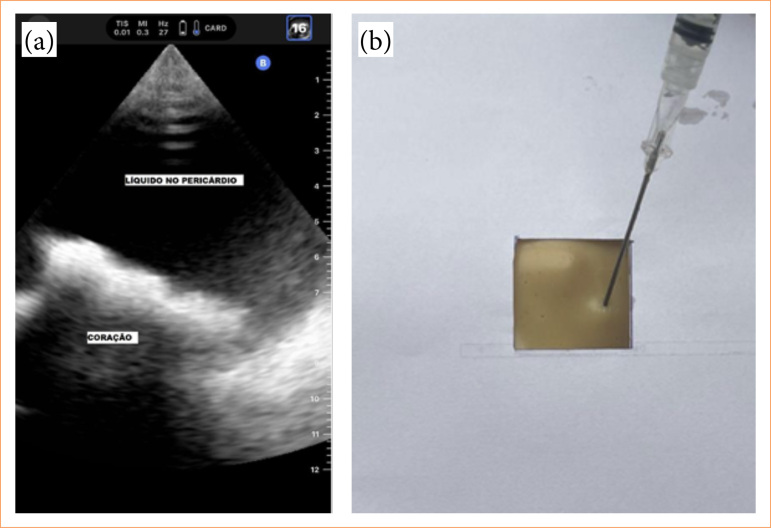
Puncture test of the finished structure, using a portable ultrasound, demonstrating the internal visualization of the assembly, and the external appearance of the puncture area.(a) Ultrasound imaging sample, (b) puncture site and retrieval of the liquid.
